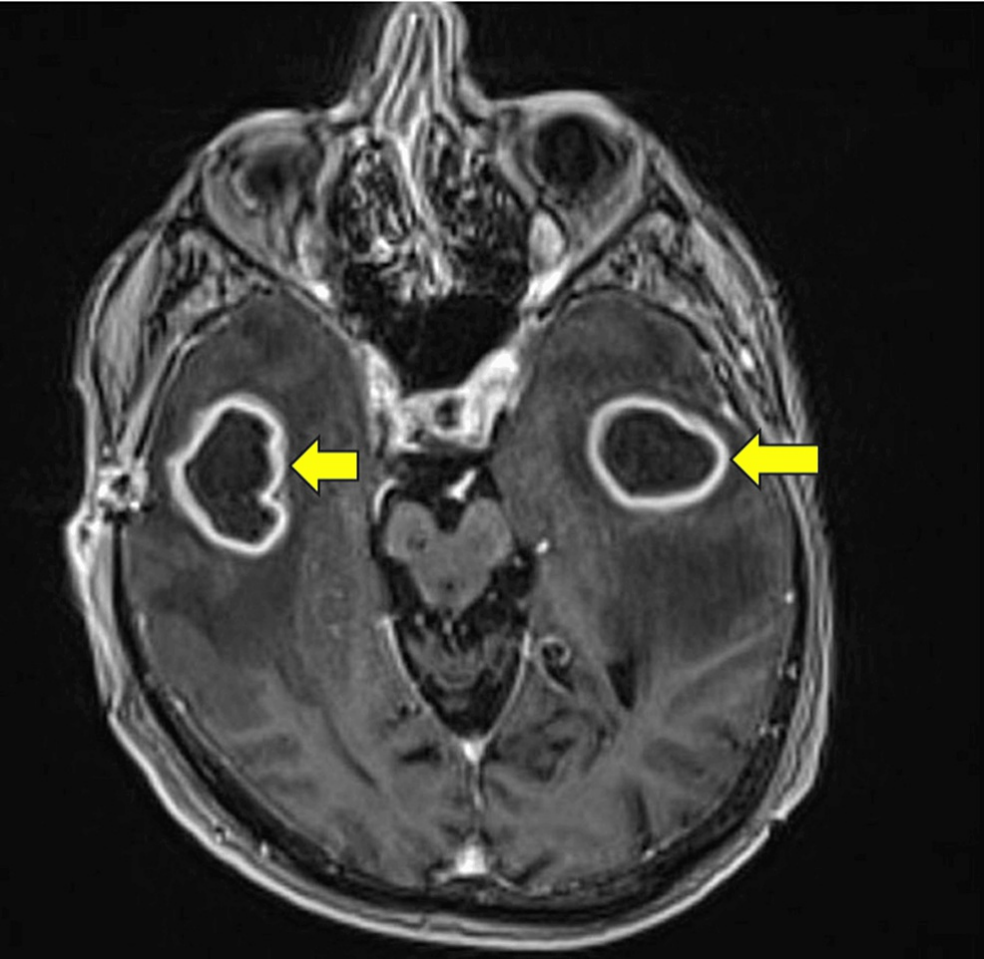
Cross-sectional view Contrast-enhanced magnetic resonance imaging of the head shows ring-enhancing lesions (yellow arrows) in bilateral temporal lobes with a central hypointense area (cystic) and a thick rim, along with surrounding regional edema of the temporal lobes.
